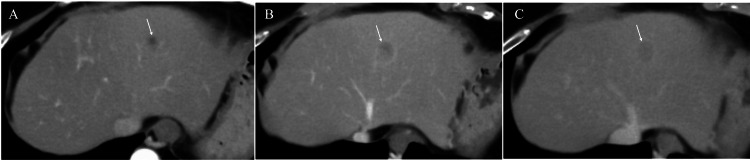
Computed tomography (CT) findings (A) CT showed no clear enhancement of the mass (arrow) on arterial phase images. (B) CT showed a very weak rim enhancement (arrow) on portal phase images. (C) CT showed retained nominal enhancement (arrow) on late-phase images.
